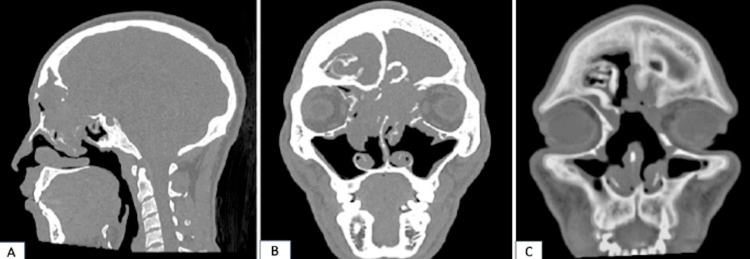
Preoperative and postoperative CT scans of the paranasal sinuses A. Sagittal view; B. Coronal view, soft tissue window displaying an ill-defined lesion anterior, posterior ethmoids, and frontal sinus causing bony erosion/remodeling and intracranial extension; C. Three months postoperative CT scan of the paranasal sinuses with a minimal soft tissue lesion seen in the left frontal sinus
